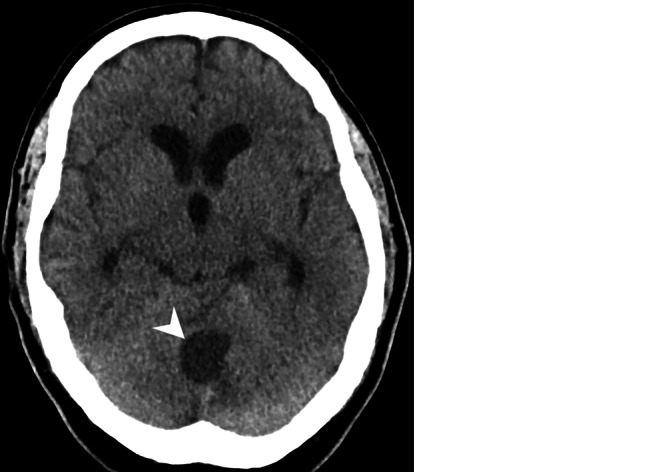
Axial CT image of the head in soft tissue settings obtained upon admission showed a cystic lesion within the cerebellum (*arrowhead*). Note the distended lateral and third ventricles, indicating obstructive hydrocephalus
